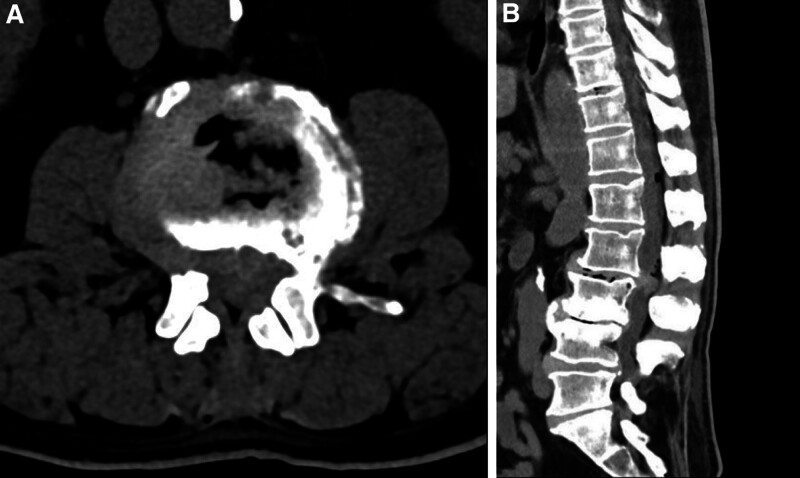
(A) Axial and (B) sagittal CT images showing disc herniation with bilateral radicular stenosis at L2/3, L4/5, and L5/S1, and stenosis of the L2/3 spinal canal. Multiple intervertebral disc pneumatization. CT = computed tomography.
